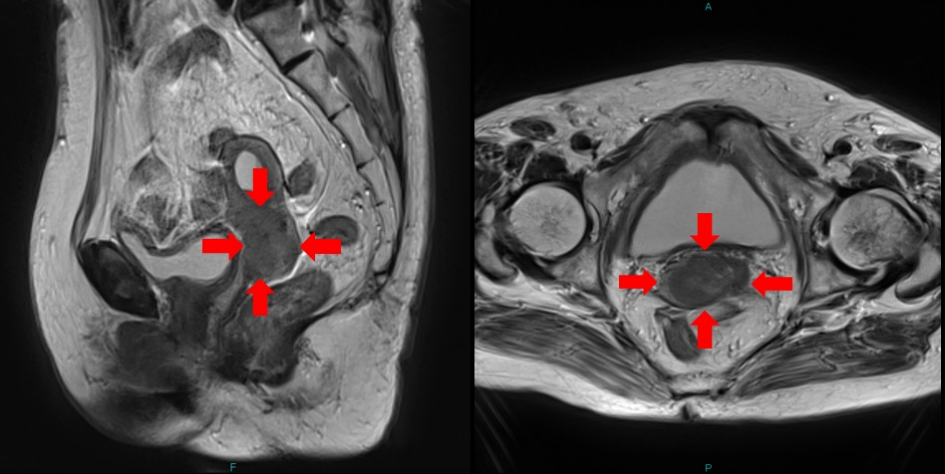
MRI of Case 1. T2WI (T2 weighted image) of Case 1 before SBRT. The tumor is shown by the red arrows. (MRI: magnetic reasonance imaging)
